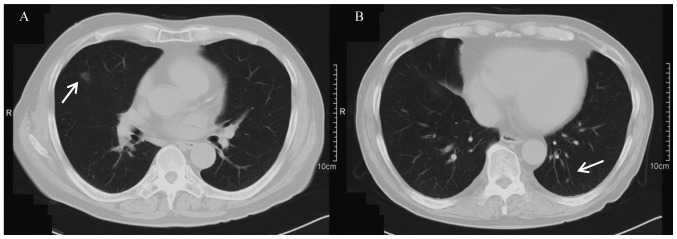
Chest computed tomography following three courses of therapy. (A) The tumor in the right lung has decreased in size to ~10 mm in diameter (arrow); and (B) the tumor in the left lung has decreased in size to ~3 mm in diameter (arrow).
